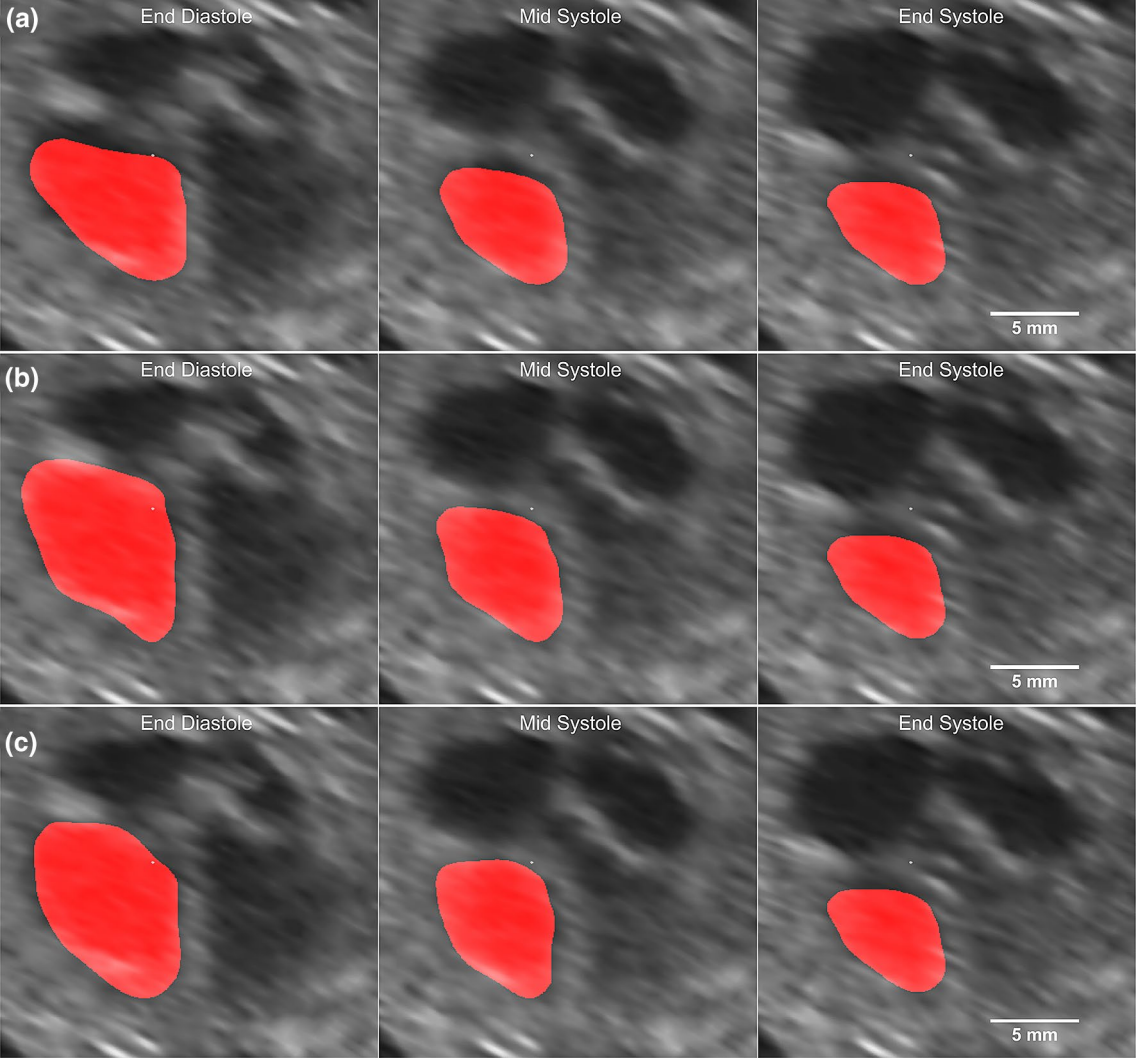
Fetal1 right ventricle geometry overlaid on top of ultrasound at different time points for (a) consistency corrected data without additional input, (b) consistency corrected data with additional vector inputs, (c) manually segmented geometry.
